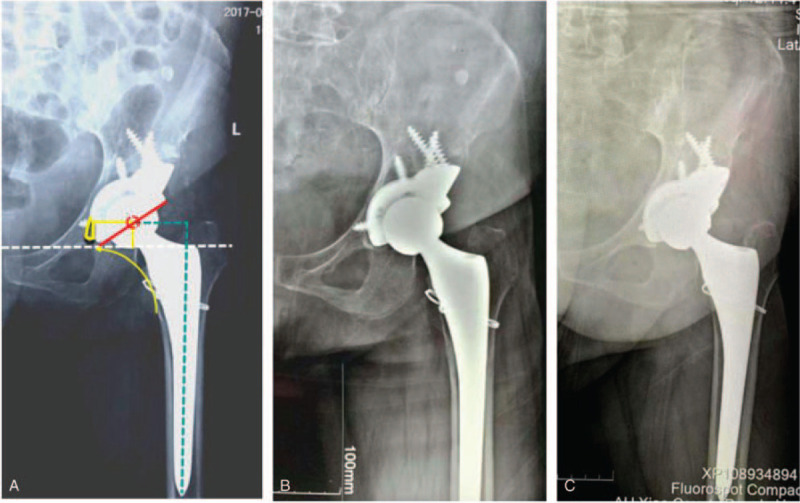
(A) Postoperative X-ray. (B) X-ray at 6-month follow-up. (C) X-ray at 12-month follow-up.
